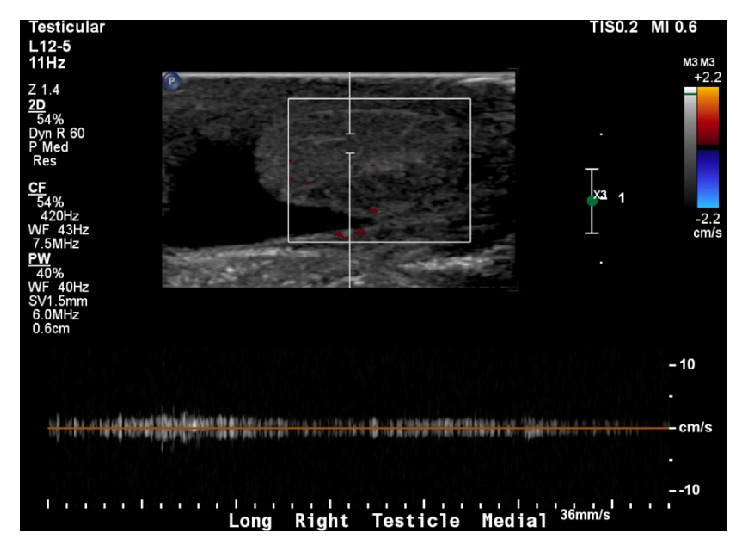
Doppler image of right testis. Normal testicular morphology was noted and no intratesticular masses were identified. Normal blood flow to the testicle was demonstrated.
